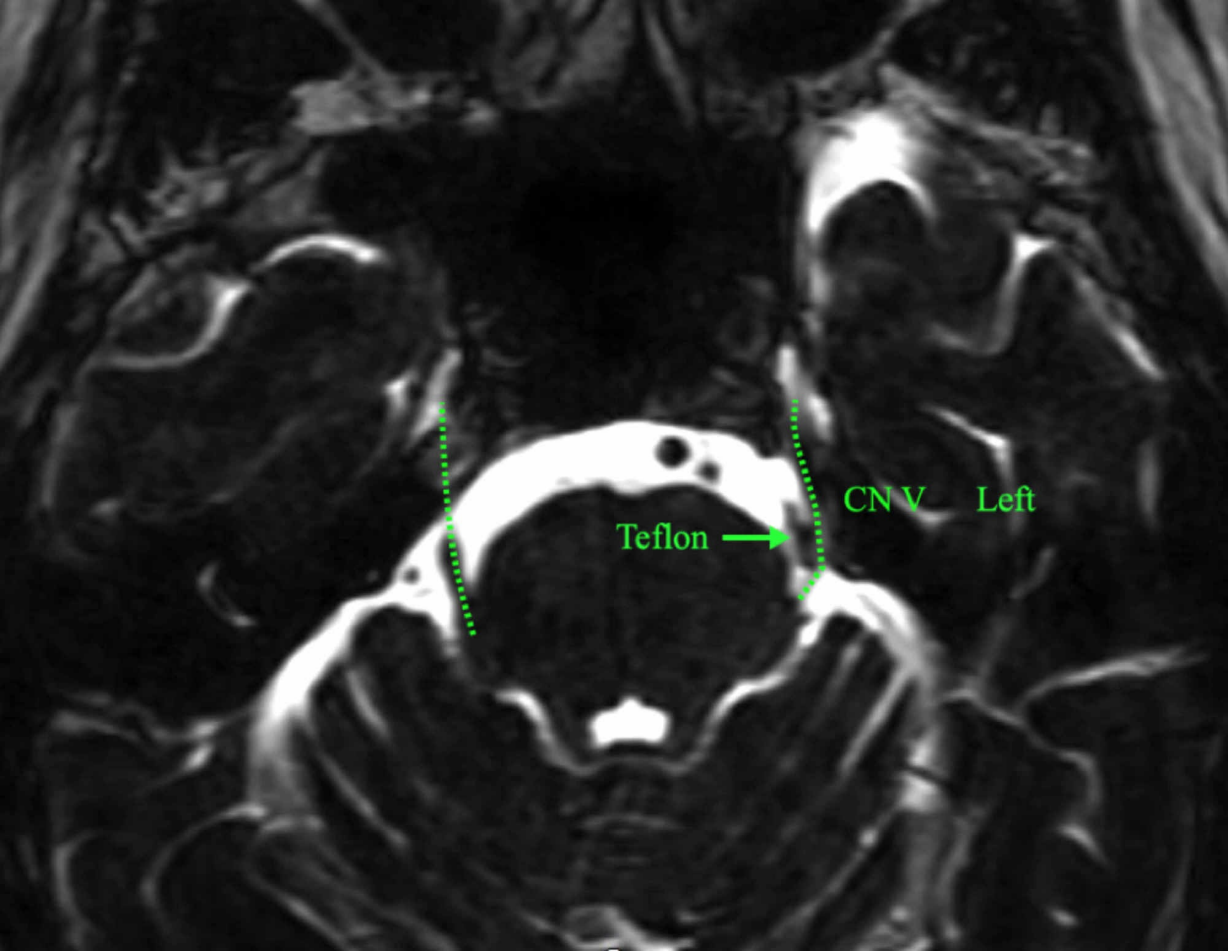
MRI of the brain shows posterior kinking of the left trigeminal nerve (CN V) with the padding seeming to be in place.
